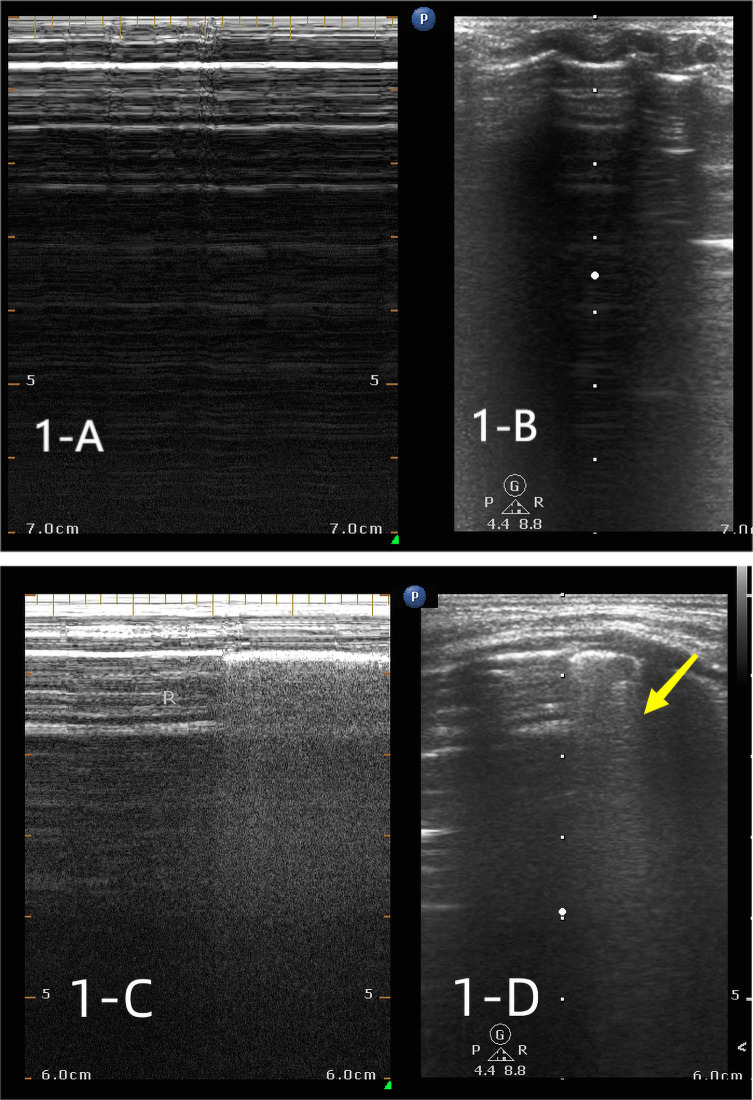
Lung ultrasound in neonatal pneumothorax. (A) M-mode showing stratospheric sign. (B) B-mode image representing the anterior chest wall, where absent lung sliding was dynamically observed in real-time during the examination. (C,D) Post-drainage reappearance of lung sliding and B-lines (yellow arrows).
